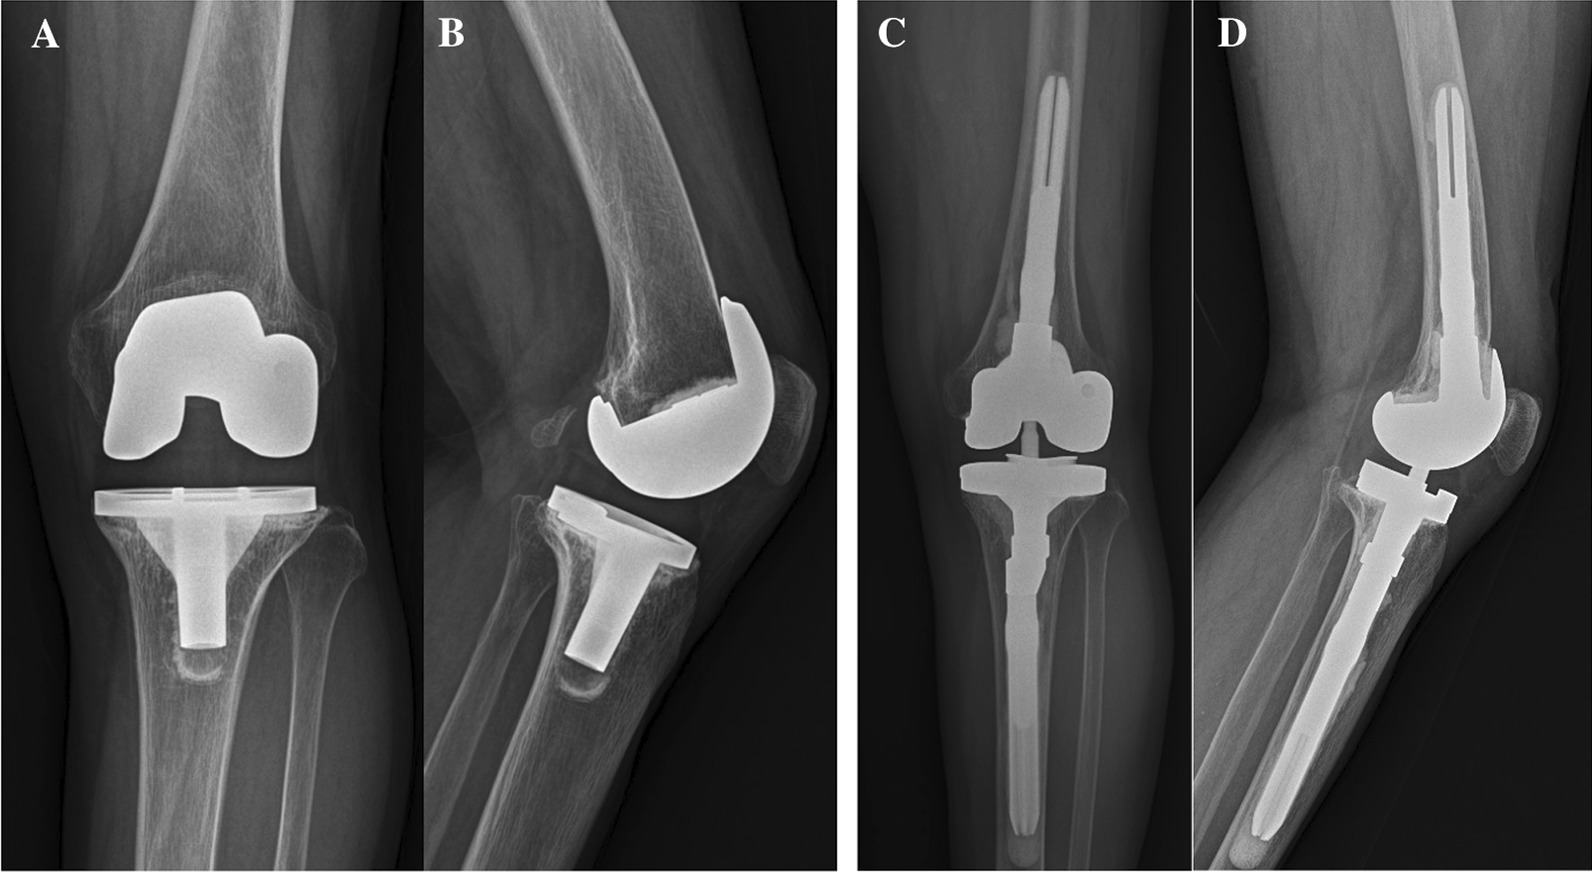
A, B Aseptic loosening of tibial component was observed on the left knee anteroposterior and lateral radiographs 10 years after primary TKA in a 77-year old woman. C, D One-stage revision TKA using a hybrid cementation technique with press-fit cementless stem was performed. Since anatomical malalignment in which the center of the tibial canal was eccentric was observed, a press-fit long stem of tibia (14 × 110 mm) with a 6 mm offset adapter was used
